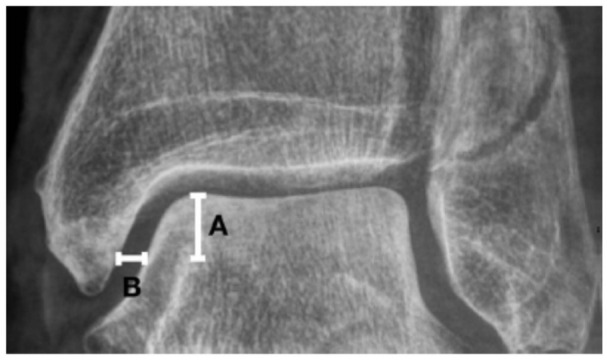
Method of obtaining medial clear space measurements from mortise view weightbearing radiographs. Line A is drawn from the top of the medial shoulder of the talar dome and 5.0 mm in the plantar direction. Medial clear space is measured as the distance between the talus and the medial malleolus on a line parallel to and 5.0 mm below the talar dome (line B).
